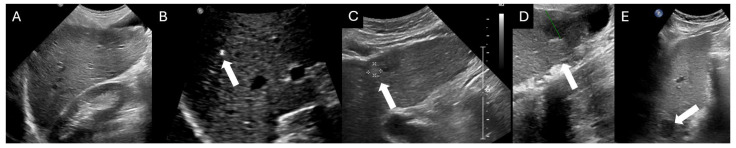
Ultrasound of the liver in five patients with chronic liver disease with respective US LI-RADS scores. Image (A) demonstrates no focal abnormality and corresponds to a US LI-RADS score of 1. Follow-up US needs to be performed every six months. Images (B,C) demonstrate subcentimeter observations measuring 0.29 cm and 0.8 cm respectively (arrows), which are assigned a US LI-RADS score of 2. Short-term follow-up examinations need to be performed to look at the evolution of these observations. Images (D,E) demonstrate observations larger than 1 cm (arrows), which are assigned a US LI-RADS score of 3. These need to be characterized further on multiphasic CT or MRI.
